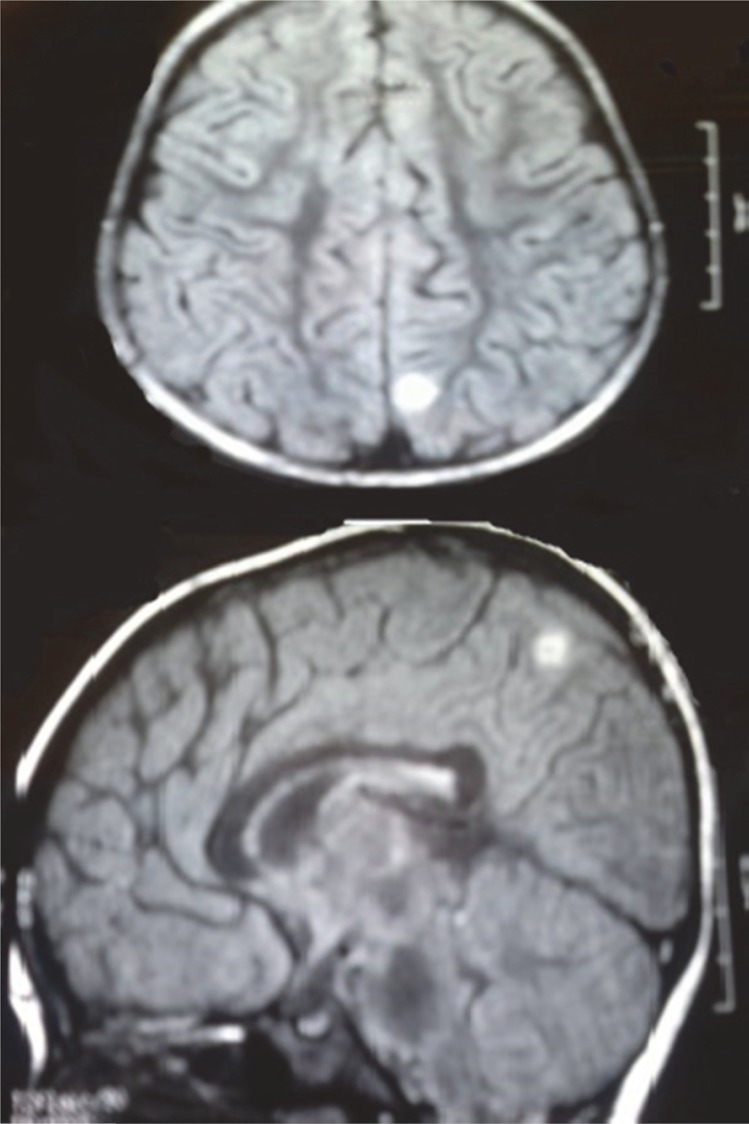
Round hyperintense lesion at left parasagitalparieto-occipital area in brain MRI
